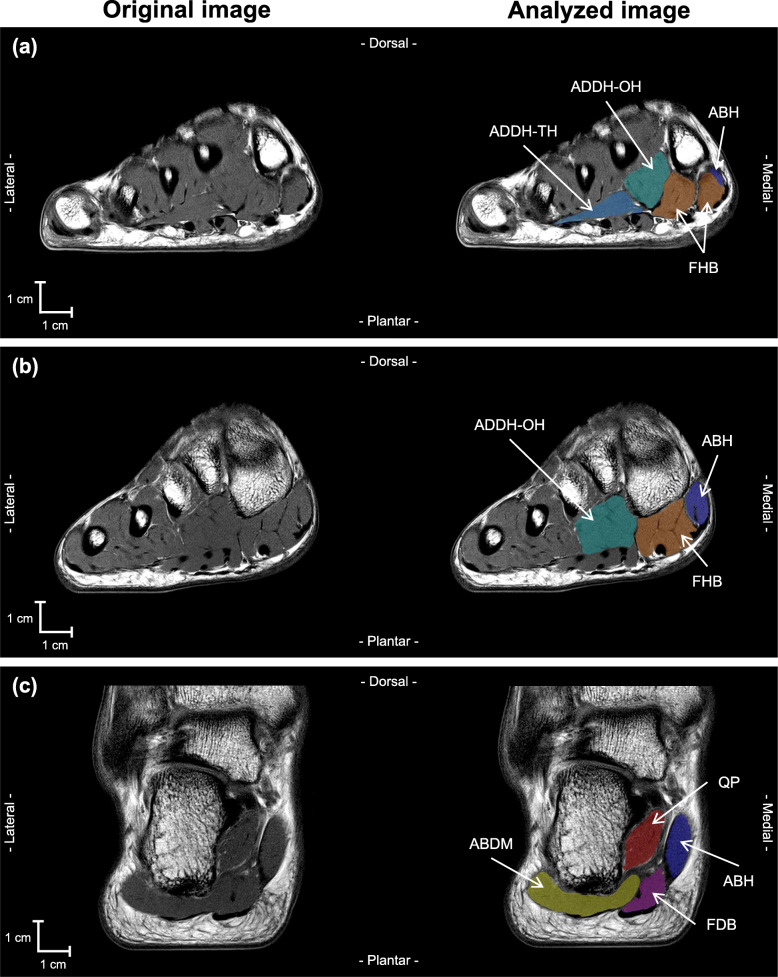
MRI images of right foot. Original and analyzed MR images are shown at the left and right side, respectively. Panel a, b, and c indicate MRI cross-sectional images obtained at the level of the mid-shaft of the first metatarsal of the foot, tarsometatarsal (Lisfranc) joint, sustentaculum tail, respectively. ABDM (abductor digiti minimi), ABH (abductor hallucis), ADDH-OH (adductor hallucis oblique head), ADDH-TH (adductor hallucis transverse head), FDB (flexor digitorum brevis), FHB (flexor hallucis brevis), QP (quadratus plantae) were manually segmented
